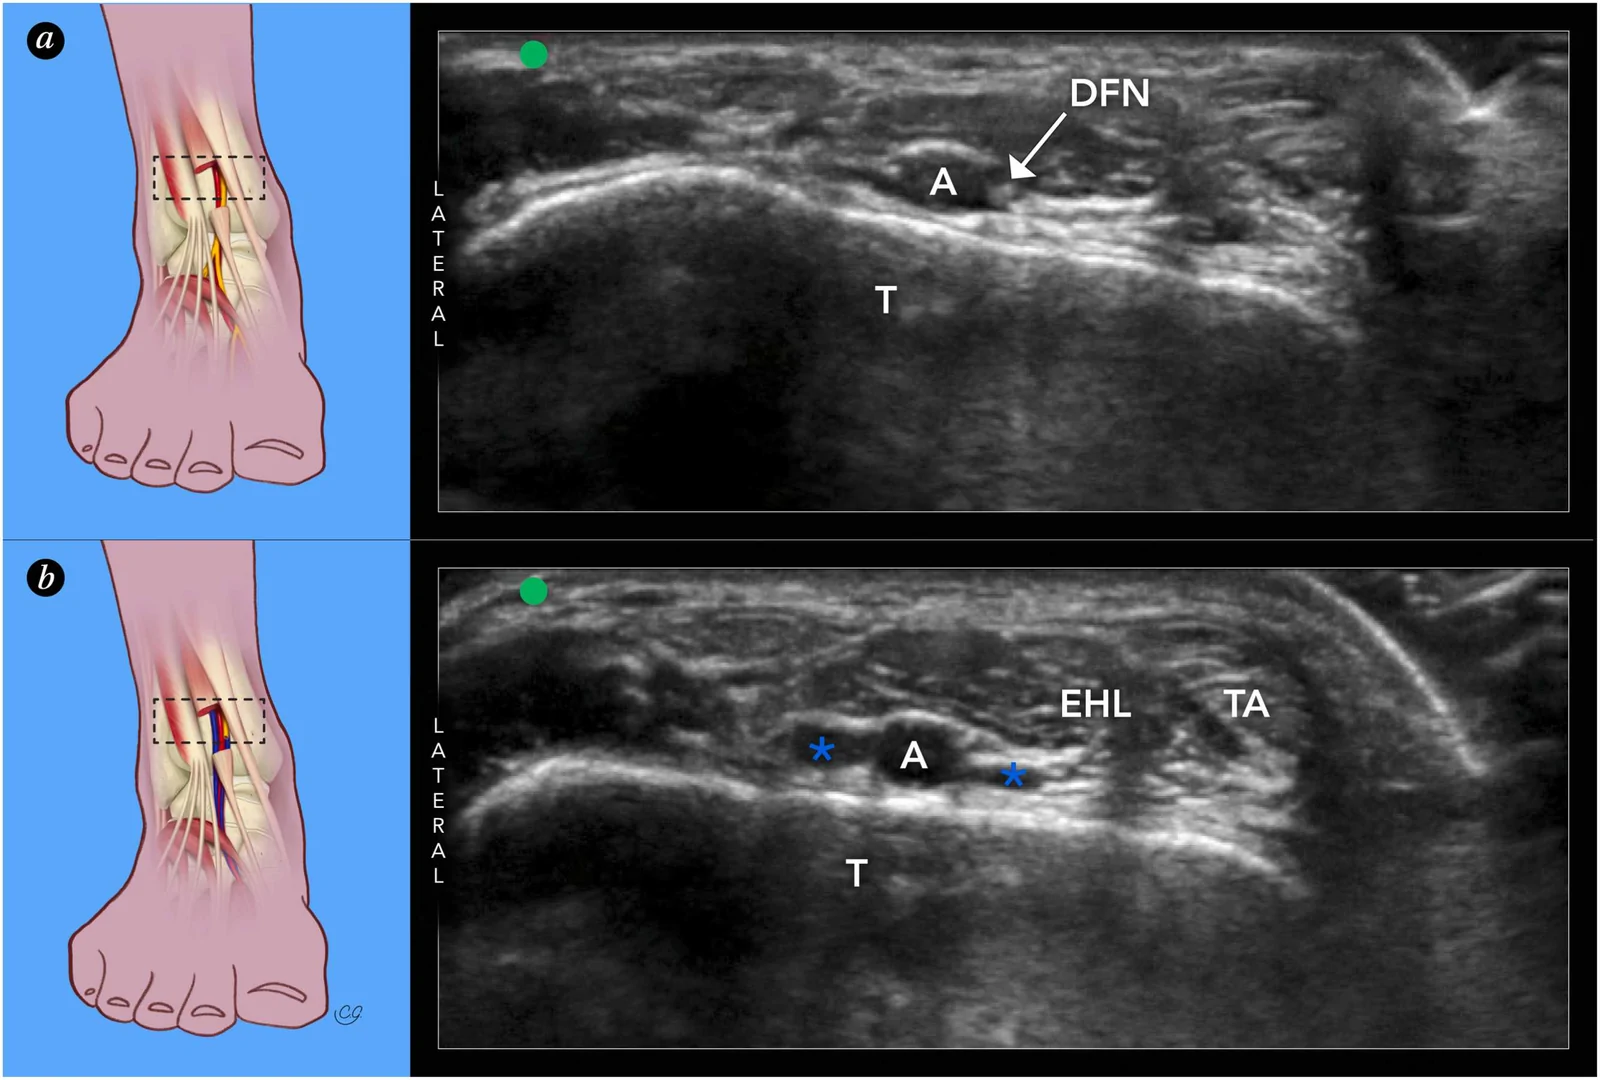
(a) Ultrasound image and probe position for the deep fibular nerve block. The surrounding veins and the dorsalis pedis artery are visualized after release of probe pressure in (b). Please note that the neurovascular bundle is typically lateral to the tibialis anterior (TA) tendon and deep to the extensor hallucis longus (EHL). The latter structure can be easily identified by plantarflexion of the big toe. DPA: dorsalis pedis artery, DFN: deep peroneal nerve, T: tibia, *: veins. Reproduced with permission from Girón Arango Medicine Professional Corporation.
